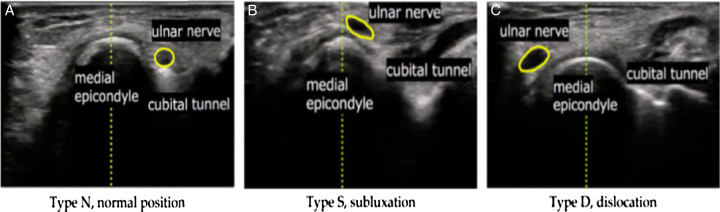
Short-axis images at the cubital tunnel using ultrasonography. (A) Type N, normal position (ulnar nerve was into the cubital tunnel); (B) Type S, subluxation (ulnar nerve was on the tip of the medial epicondyle); and (C) Type D, dislocation (ulnar nerve exceed and positioned anteriorly to tip of the medial condyle)^7^.
